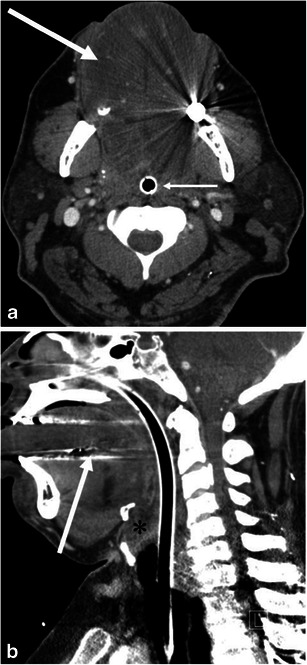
A 49-year-old male with ACE inhibitor-induced angioedema. a The axial CE-CT shows diffuse enlargement of the tongue with decreased attenuation due to oedema (*large arrow*). *Small arrow* indicates the endotracheal tube. b Sagittal CE-CT shows glossomegaly (*arrow*) and marked laryngeal oedema (*asterisk*). The endotracheal tube is inserted by the trans-nasal approach because of marked glossomegaly
